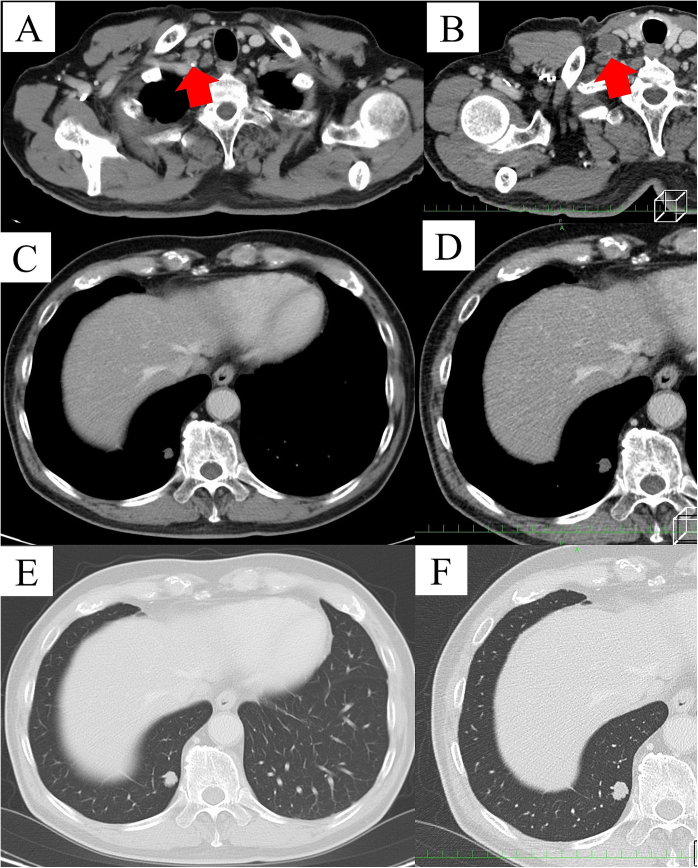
(A-B) Thoracic computed tomography (CT) images revealing an enlarged supraclavicular lymph node with low attenuation and poor enhancement (red arrow). (C-F) CT images showing a well-defined solitary hypoattenuated tumor with minimal enhancement in the right lower lobe. (For interpretation of the references to colour in this figure legend, the reader is referred to the web version of this article.)
